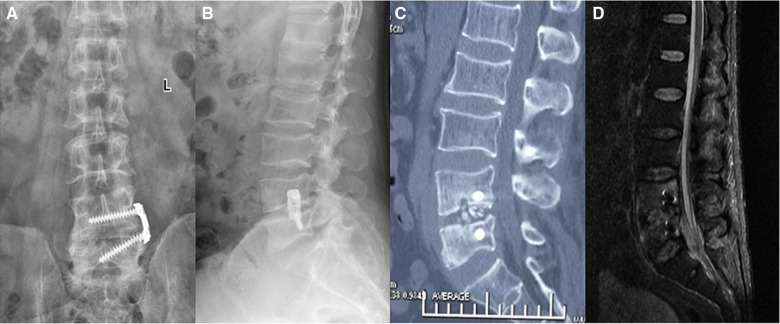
12 months postoperatively. X-rays (A,B), CT (C), and MRI (D) showed that the internal fixation position was good, the bone graft was fused, and the abnormal signals in the lumbar vertebral body, spinal canal, and psoas major muscle had disappeared.
